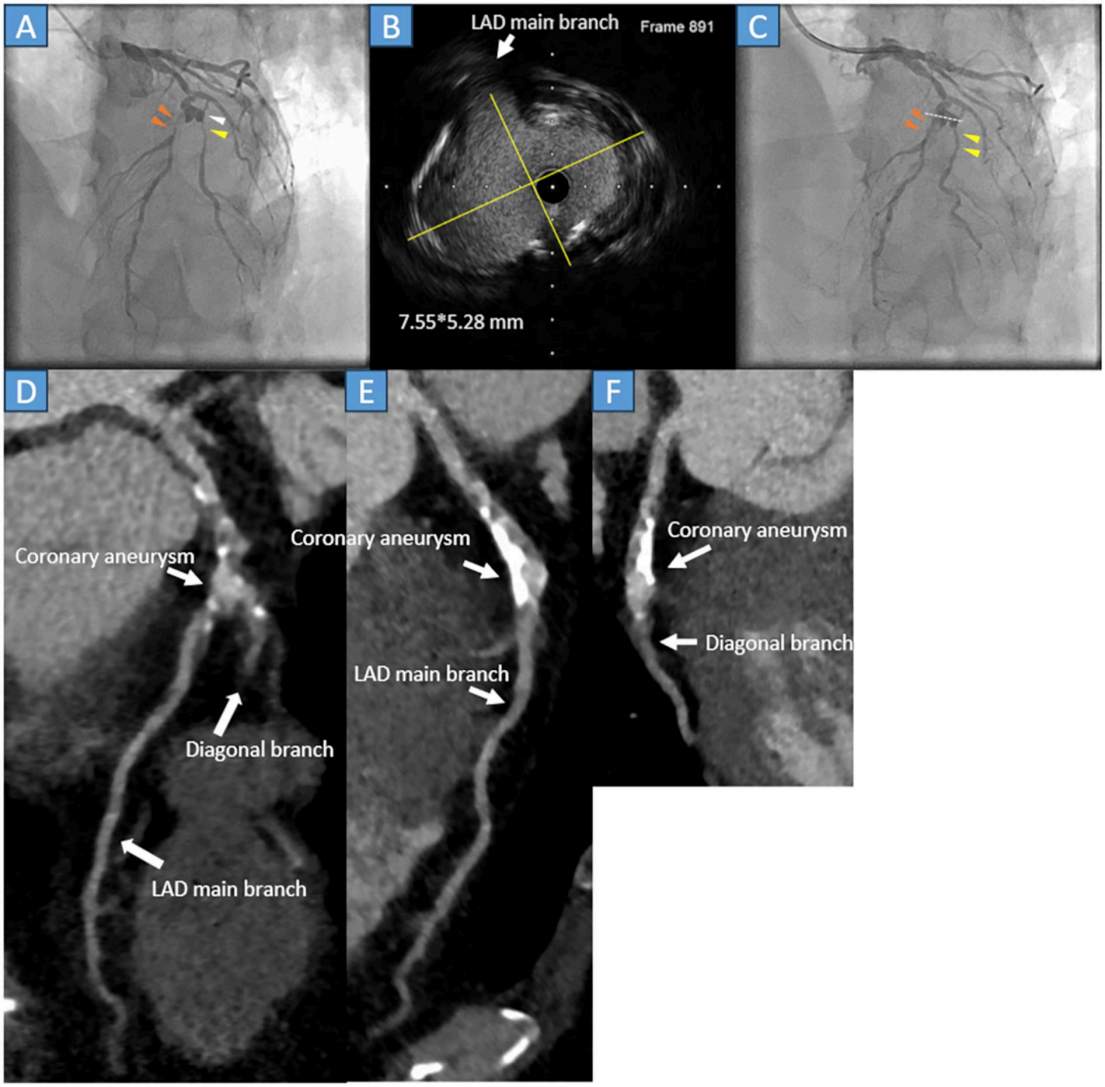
(A) Coronary angiography reveals severe stenosis at the middle segment of the left anterior descending artery (LAD) (orange arrowheads) and the second diagonal branch (yellow arrowhead), just after the aneurysm formation (white arrowhead). (B) The cross-sectional image of the intravascular ultrasound (IVUS) detects a 7.55*5.28 mm (yellow lines) mild calcified oval-shaped coronary aneurysm. (C) The LAD (orange arrowheads) and second diagonal branch (yellow arrowheads) were successfully treated with the drug-coated balloon. The white dots in the angiography correspond to the cross-sectional IVUS image. (D) Cardiac computed tomography showing patient LAD and secondary diagonal branches with calcification. (E) The LAD is patent with atherosclerotic change and calcification. (F) The second diagonal branch is patent, and the main branch of the LAD is severely calcified.
